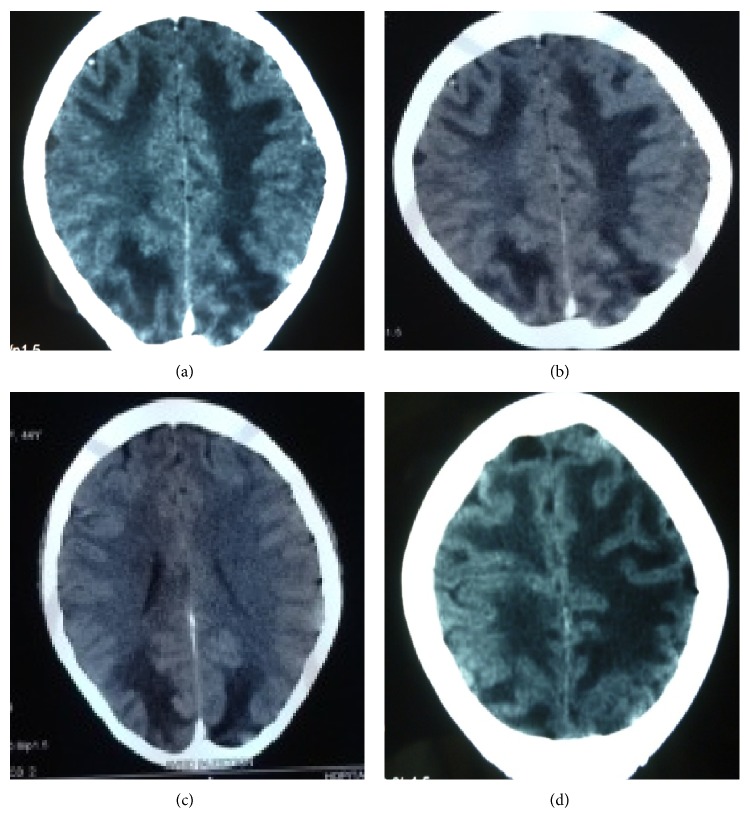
Brain CT scan with contrast shows subcortical hypodensities in the frontoparietooccipital region of white mater bilaterally and symmetrically without contrast enhancement.
